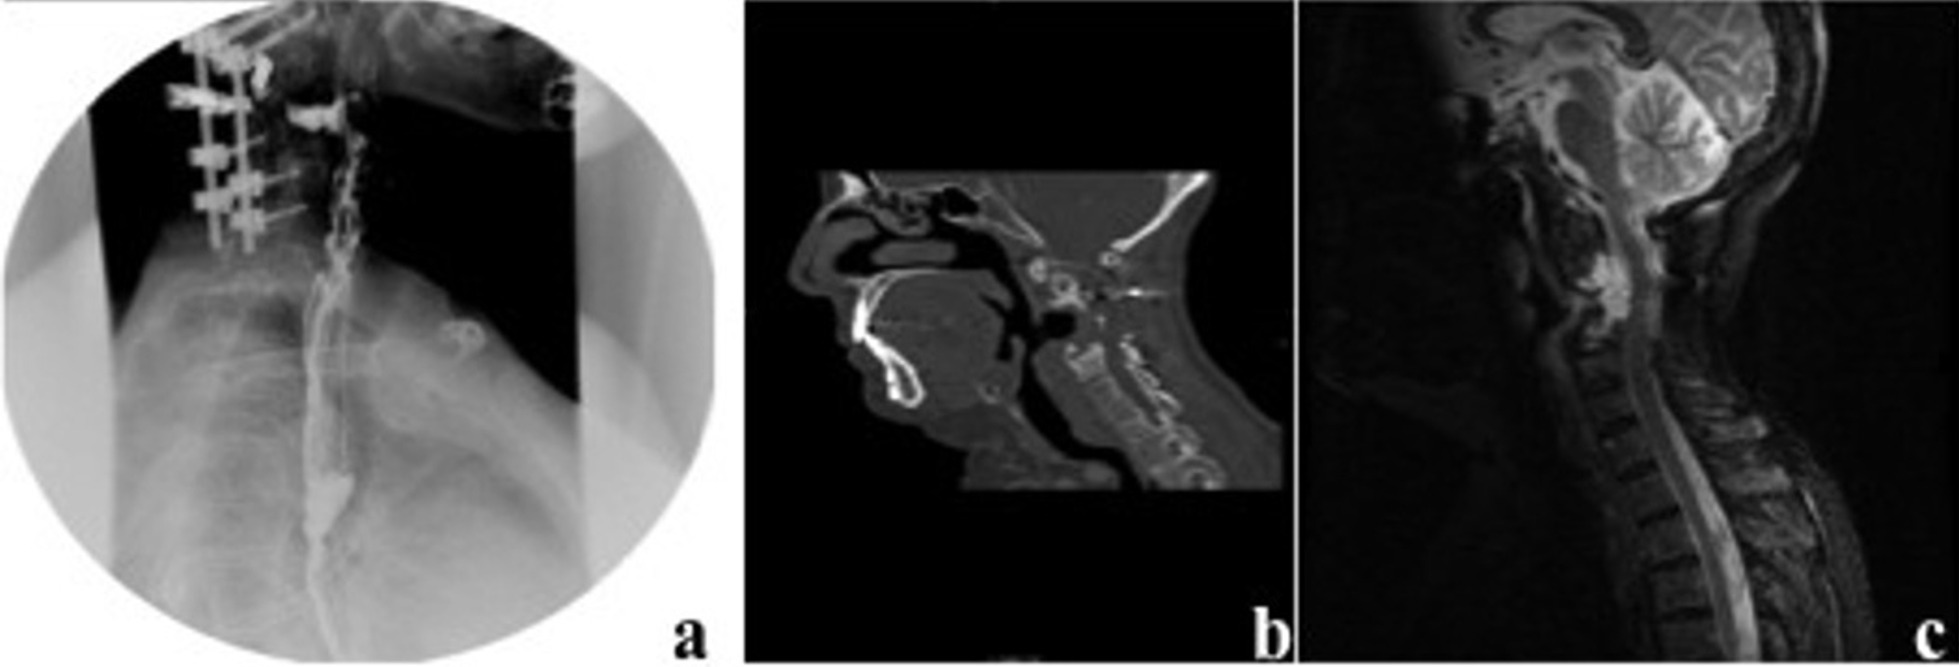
a Esophagram test and b CT scan show the decubitus ulcer in the posterior wall of the oropharynx; c magnetic resonance image shows tumor relapse
